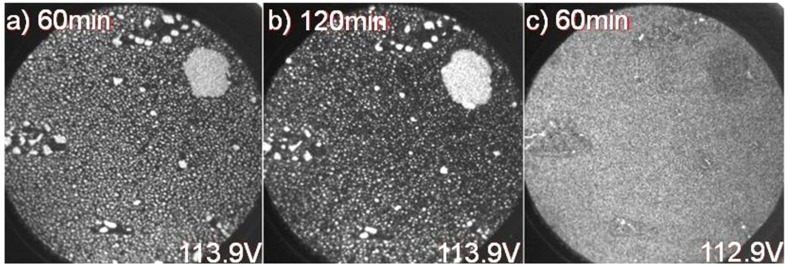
XPEEM images recorded, at a FOV = 25 µm and hv = 150 eV, from the Na 2p component at 0 eV relative binding energy after (a) 60 and (b) 120 min. of soft X-ray exposure; (c) XPEEM image from the other Na 2p component located at around 1.2 eV and after 60 min of soft X-ray exposure.
